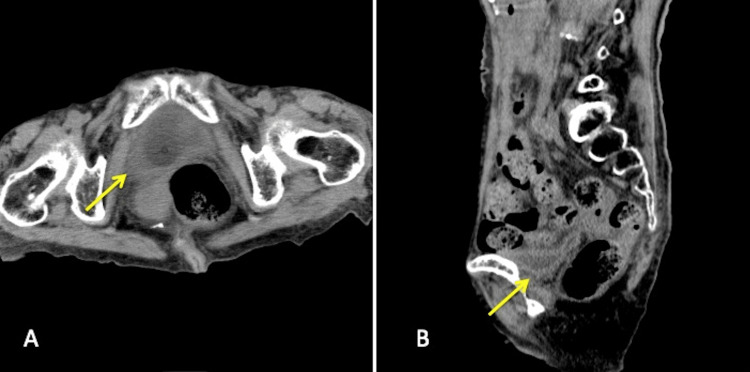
Non-contrast computed tomography performed on day 18 A: Axial image. Bladder wall emphysema was resolved (arrow). B: Sagittal image. Pneumoperitoneum was resolved (arrow).
